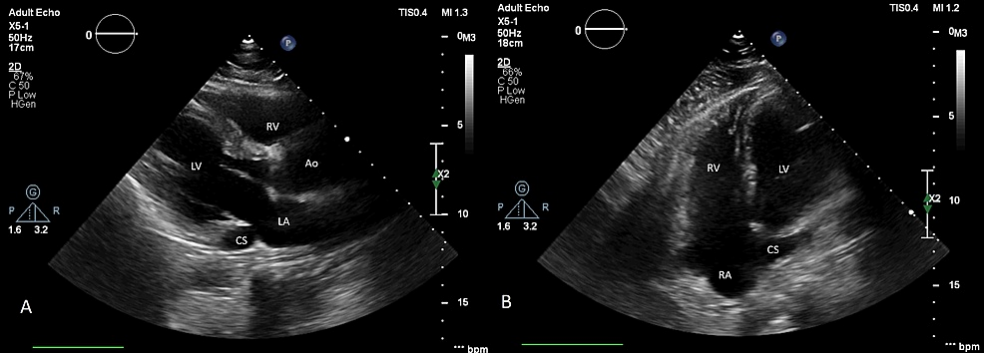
2D transthoracic echocardiographic images: (A) parasternal long-axis view showing a dilated CS; (B) foreshortened apical view showing a dilated CS. 2D: two-dimensional, Ao: aorta, CS: coronary sinus, LA: left atrium, LV: left ventricle, RA: right atrium, RV: right ventricle.
